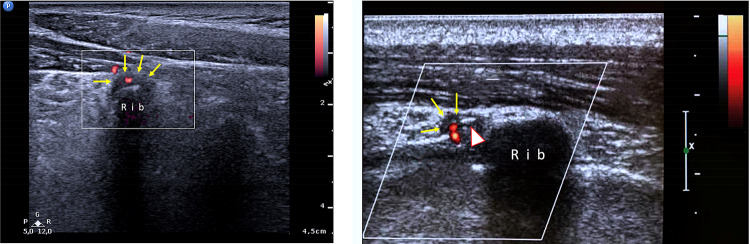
Presence of Doppler US signal in the synovial tissue (yellow arrows) in less than half of the area of the synovium (27% of cases): these findings were detected mainly in chronic inflammation; white and red arrowhead show a little calcification within the CTJ
